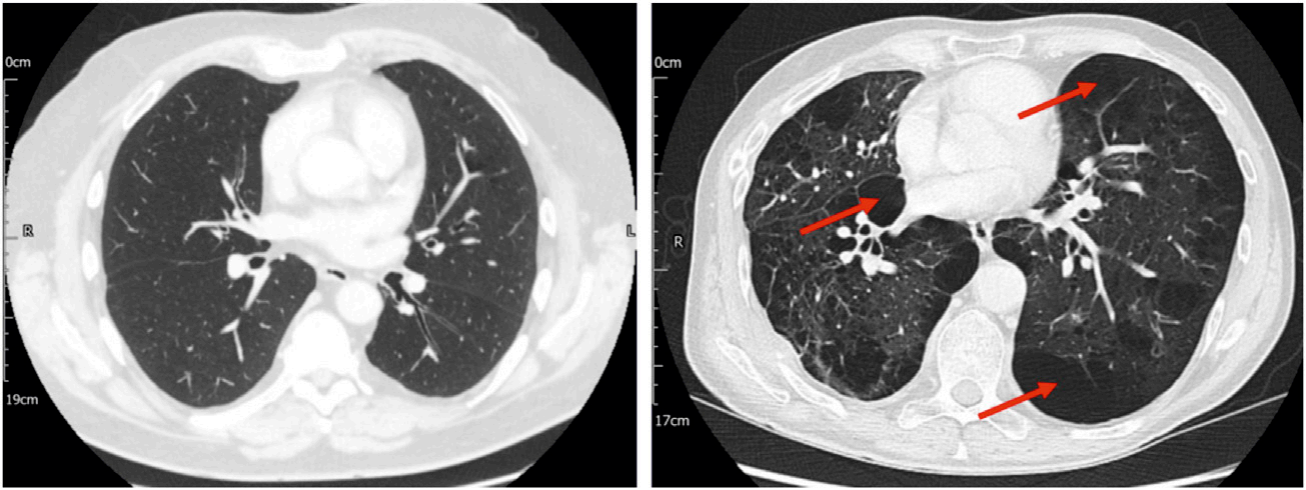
Computed tomography (CT) imaging for two patients with stage 2 COPD, one of whom had no CT evidence of emphysema (left panel) and the other of whom had significant paraseptal and centrilobular emphysema (right panel), with bullae (examples indicated by arrows). Both patients had significantly elevated σCL (0.76 and 1.06, respectively). In the patient without CT evidence of emphysema, the estimated total anatomical deadspace volume was normal (0.169 L). In the patient with CT evidence of emphysema, the estimated deadspace volume was substantially elevated (0.502 L). This highlights the possibility that lung inhomogeneity testing might not only represent a means of identifying COPD, but also of providing information about the underlying disease phenotype.
